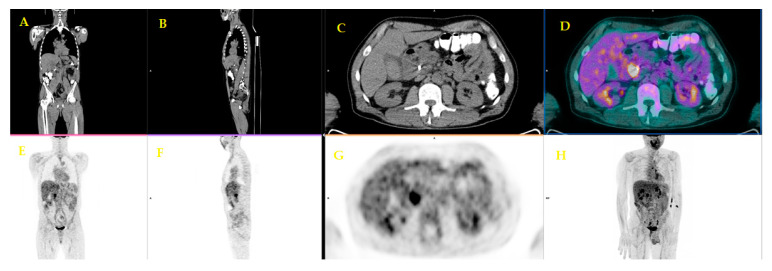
A fifty-six-year-old patient underwent staging FDG PET/CT with positive (ce)CT examination for local pancreatic lesion and equivocal for distant metastases. FDG PET/CT revealed a focal uptake on the head of the pancreas (C,D,G). (Images from Nuclear Medicine Unit, Padova University Hospital). Legend: A = Coronal image of low-dose CT; B = Sagittal image of low-dose CT; C = Axial image of low-dose CT; D = PET/CT fused image; E = Coronal image of PET; F = Sagittal image of PET; G = Axial image of PET; H = Maximum intensity Projection (MIP).
